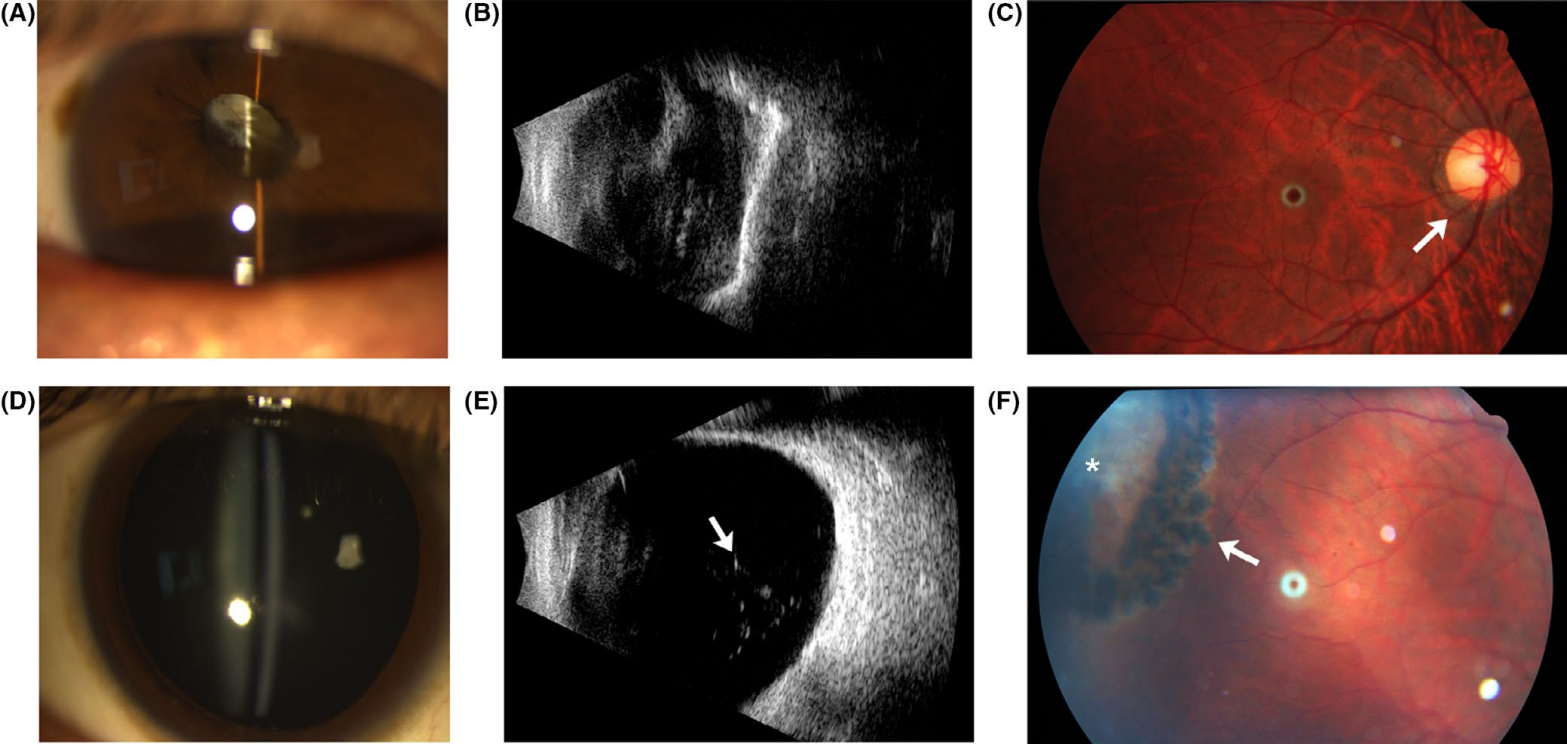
Ophthalmologic abnormalities of affected individuals of the nonsyndromic ocular STL1 family. (A, D) Anterior segment examination of IV‐15(A) and V‐3(D). (B, E)Vitreous ultrasound inspection of IV‐15(B) and V‐3(E). White arrow: dot opacification. (C, F) Fundal examination of V‐3. White arrow in (C): myopia arc. White arrow in (F): laser spots. Asterisk: retinal tear. Because IV‐15 was blind due to a retinal detachment, the segment examination was performed without pupil dilation and fundal examination was not performed
